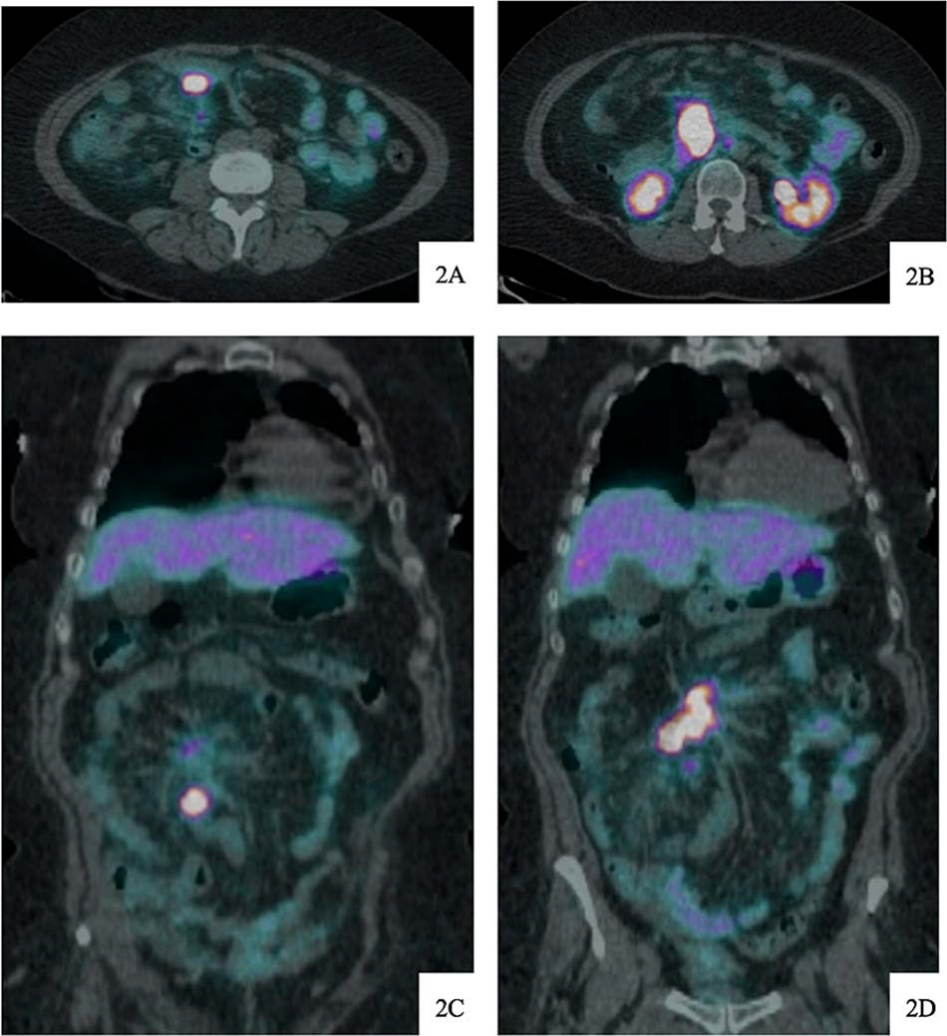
DOTATATE positron emission tomography-computed tomography scan at time of diagnosis in November 2017 revealed a small bowel primary tumor in the right lower quadrant consistent with terminal ileum (A) as well as the periduodenal mass near the root of the mesentery (B). Coronal reformatting redemonstrates the primary tumor (C) and mesenteric mass (D).
